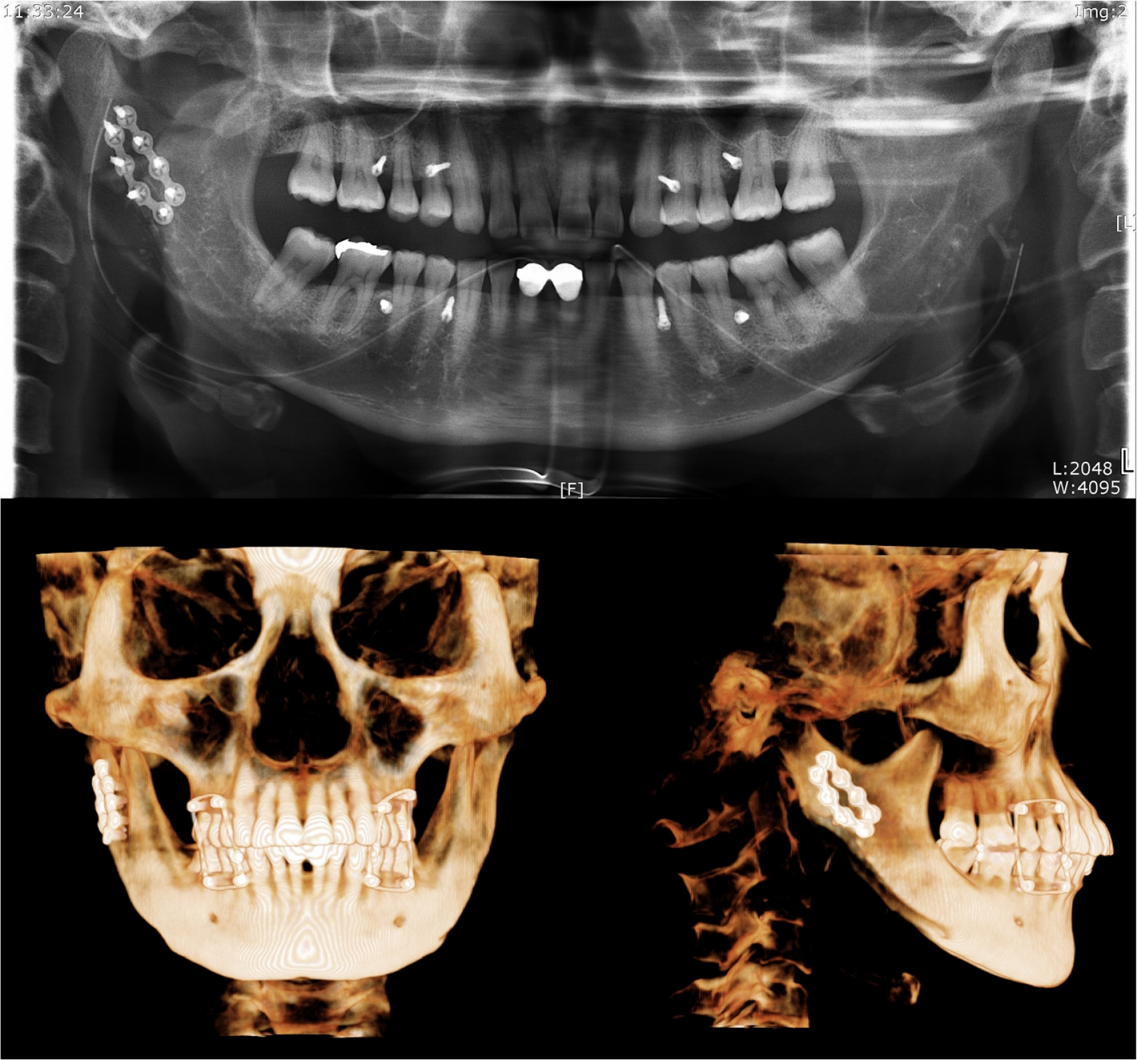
Immediate postoperative: panoramic radiograph (*above*) and 3D-CBCT, frontal and right lateral view, (*below*) showing the damage of posterior plate
